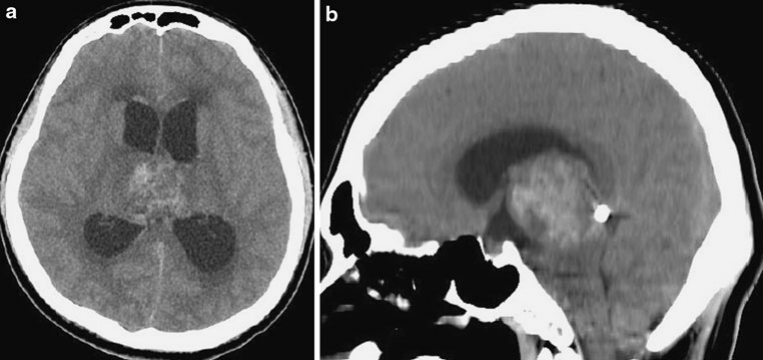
Initial plain CT scans (a axial image, b sagittal image) showing a mixed density mass with calcification in the pineal region and hydrocephalus
